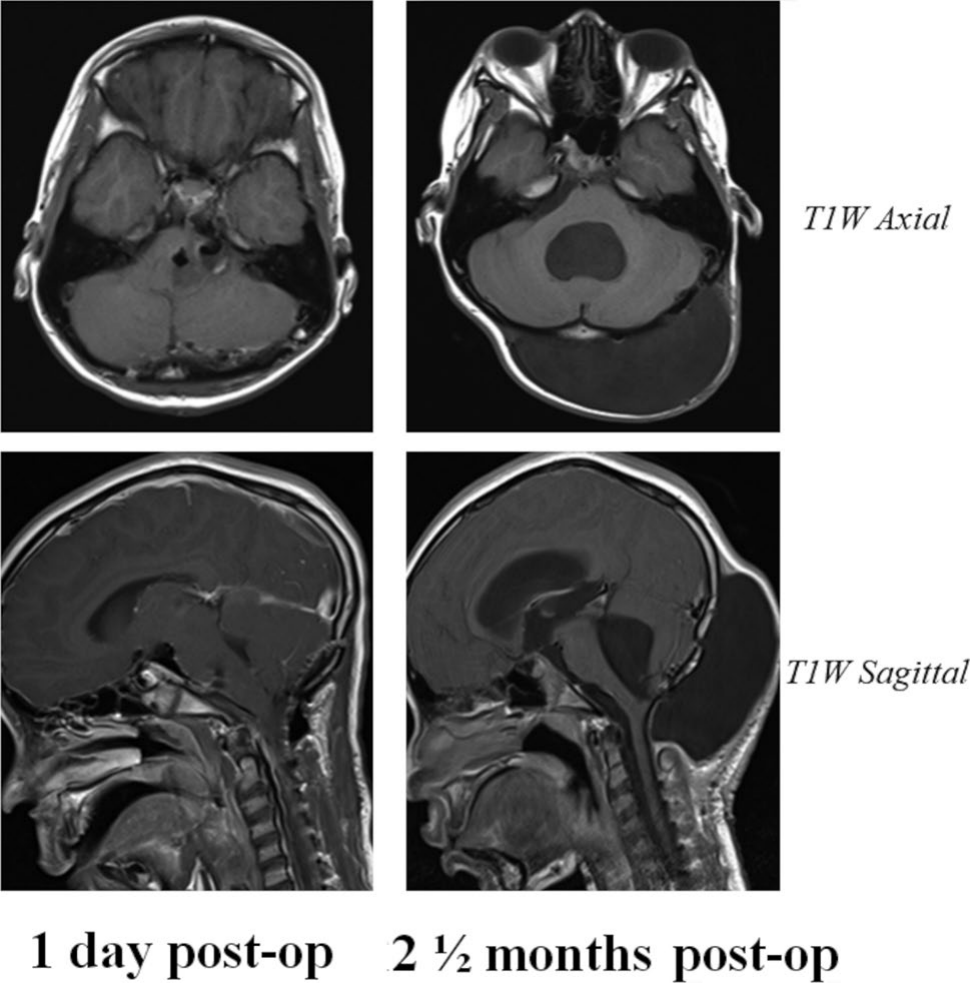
Post-operative MRI imaging showing development of occipital pseudomeningocele. Axial (*top*) and sagittal (*bottom*) T1-weighted images with or without contrast shown. Note at post-operative day 1 (*left panel*) the absence of any significant pseudomeningocele on the pre- or post-contrast T1-weighted imaging. In the weeks following surgery, a large occipital pseudomeningocele developed in association with pan-ventricular hydrocephalus. Despite an ETV attempt with good flow through the III^rd^ ventricular floor stoma (as seen on the sagittal imaging), this pseudomeningocele continued to enlarge up to 2 ½ months post-surgery with persisting ventriculomegaly (*right panel*)
